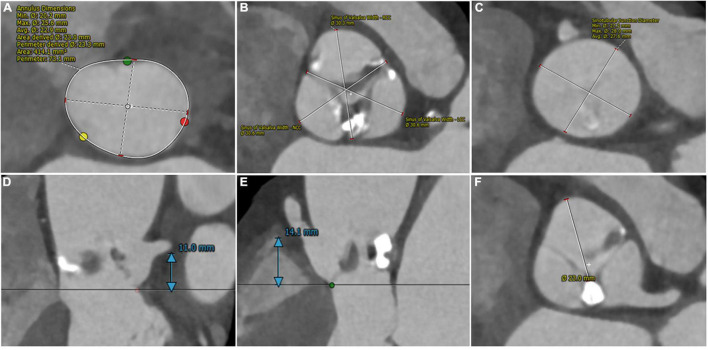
Pre-procedural computed tomography assessment of aortic root. (A) Annular perimeter: 73.1 mm and annular area: 414.1 mm^2^. (B) Left-, right- and non-sinuses of Valsalva dimensions: 31.6 mm * 30.7 mm * 30.7 mm. (C) Average sinotubular junction diameter: 27.6 mm. (D,E) The height of coronary arteries: 11 mm (left) and 14.1 mm (right). (F) The distance from the edge of the calcified nodule to right coronary ostium: 23 mm.
